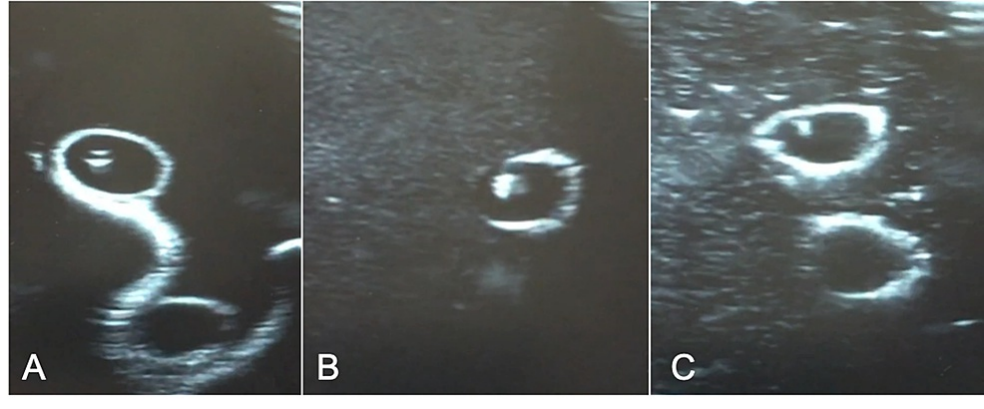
Echogenicity of models under ultrasound with the needle in the vessel A) Pure 10% ballistic gel. B) Corn starch and ballistic gel. C) Chalk and ballistic gel
